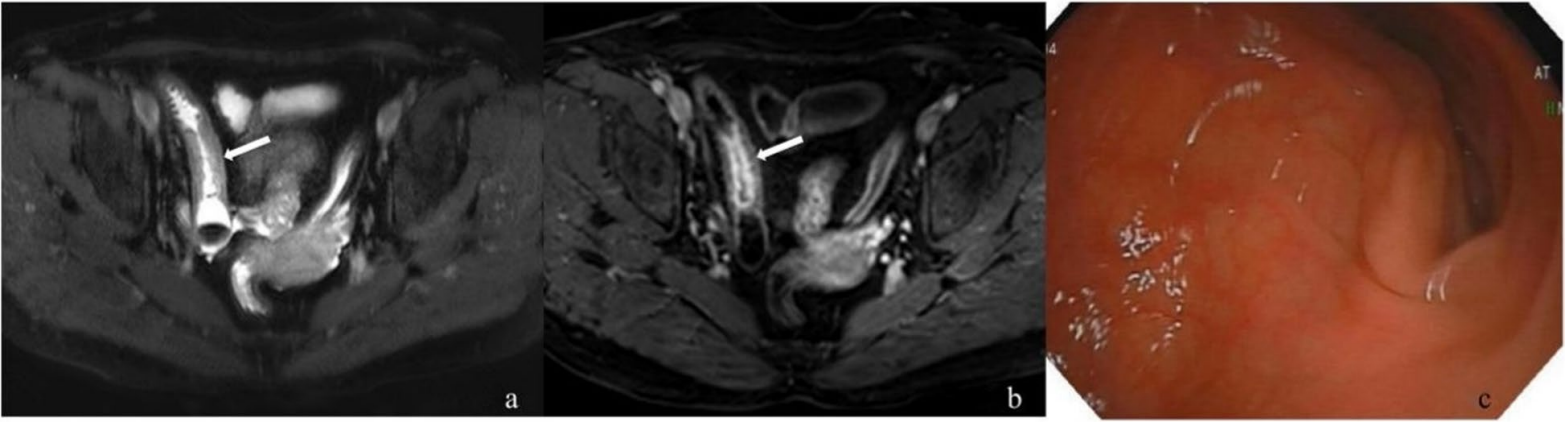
A 66-year-old woman with Crohn’s disease. a Axial T2-weighted imaging before endoscopy: *arrow* points the stenosis in the distal ileum with marked thickening of the intestinal wall and moderately high intensity. b Axial enhanced T1-weighted imaging with severe intestinal wall enhancement and mucosal enhancement (*white arrow*). c The endoscopy showed a narrow ileum, but the endoscopy could pass
